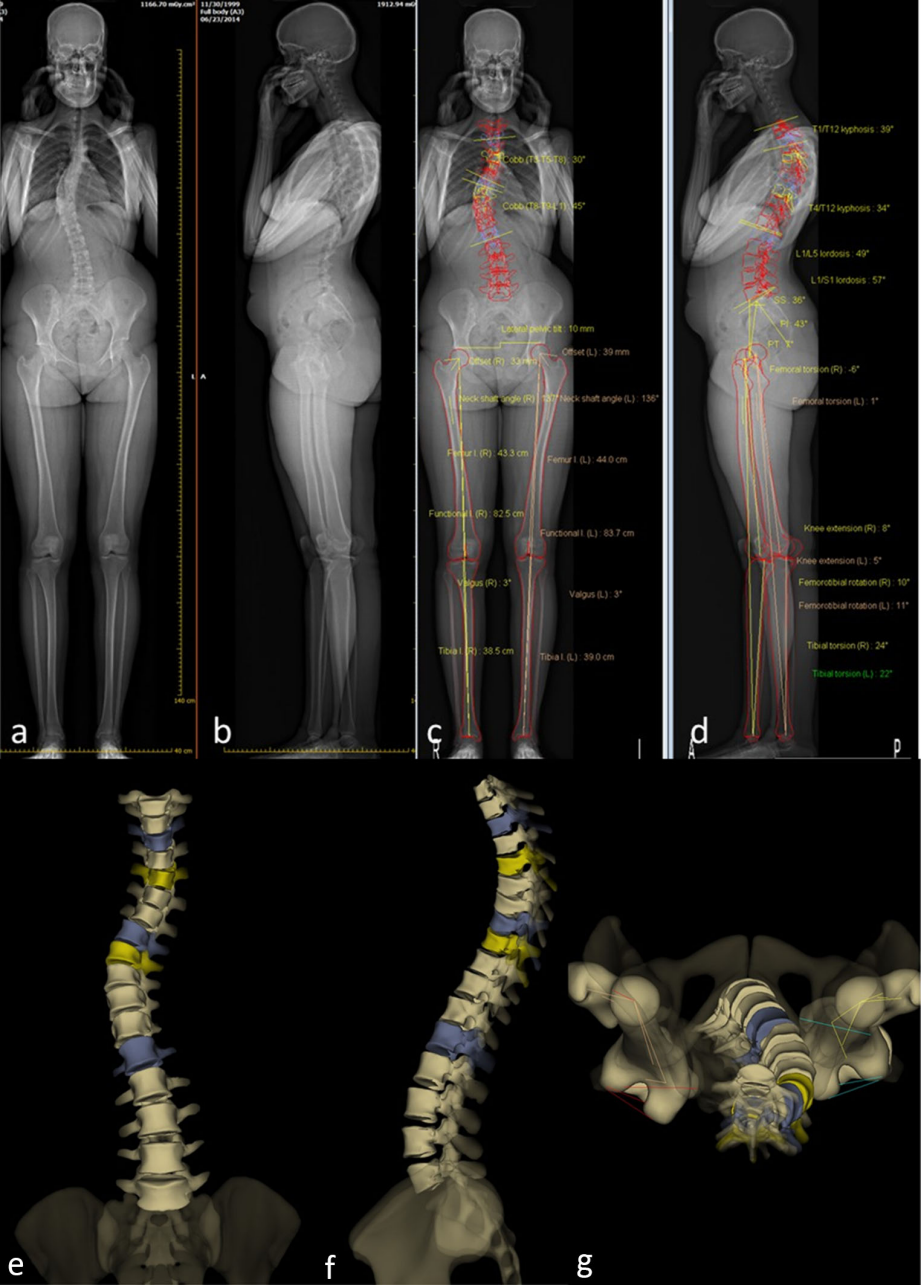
Example of 2D orthogonal X-ray images for a 15-year-old patient obtained using the EOS^®^ system, along with a 3D reconstruction
